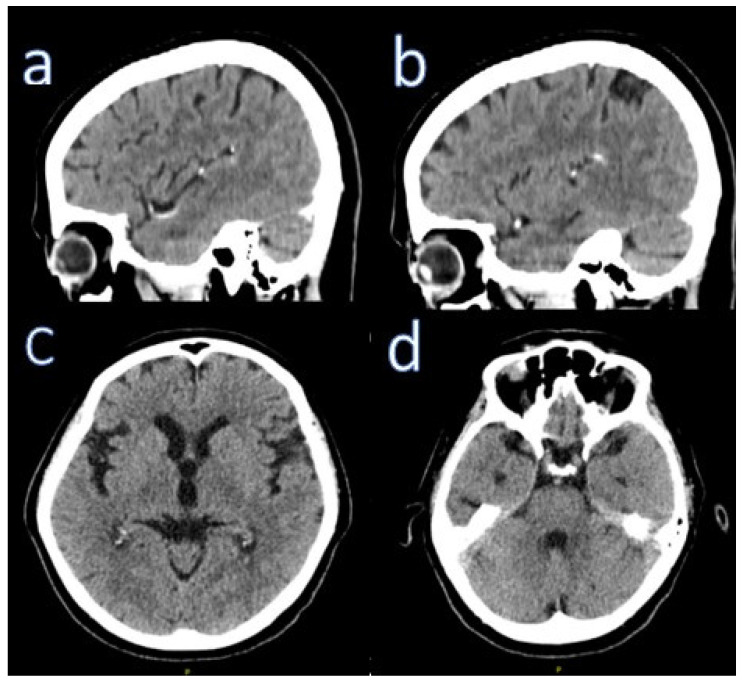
CT of Case 1 taken after first presentation in outpatients’ clinic. Sagittal (a,b) and axial (c,d) CT projections.
